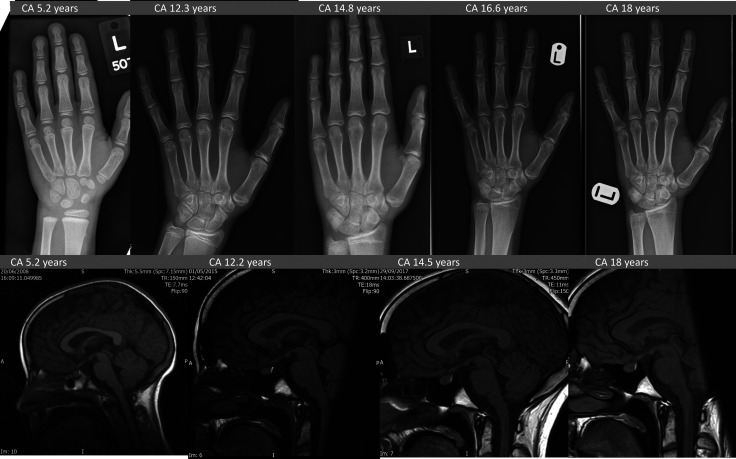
Serial pituitary MRI and bone age radiology. Upper panel shows serial bone age X-rays. (A) At diagnosis chronological age (CA) 5.2 years, bone age (BA) 6.9 years. (B) At the start of pegvisomant treatment CA 12.3 years, BA 13.5 years. (C) Stable on pegvisomant, BA advancement resolved CA 14.8 years, BA 15 years. (D) Linear growth had resumed CA 16.6 years, BA 16 years. (E) At final height CA 18 years, BA 17.6 years (ie, mature). Lower panel: 4 of 10 serial pituitary MRI scans. All demonstrate slightly bulky but not enlarged pituitary of homogenous texture and enhancement; appearance and size and unchanged throughout the 13-year period. Sagittal T1-weighted images with gadolinium contrast (F) at diagnosis, (G) at the start of pegvisomant treatment at CA 12.3 years, (H) 2.3 years after pegvisomant treatment started, (J) 5.8 years after pegvisomant treatment started; (K) at CA 18 years.
